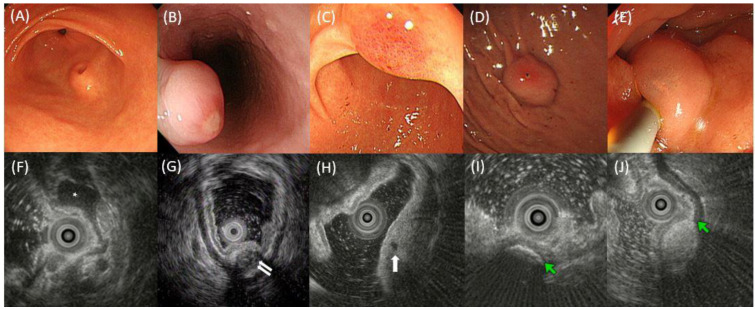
Examples of the endoscopic and EUS findings of SELs. (A) Umbilication or central dimpling, (B) erosion or ulcer, (C) erythema, (D) hemorrhagic spot, and (E) translucidity. (F) EUS image showing a homogeneous hypoechoic SEL (asterisk) with a distinct border; (G) EUS image showing an SEL involving muscle propria layer (white arrows) with heterogeneity, mixed echogenicity, indistinct border, and deep attenuation; (H) EUS image showing anechoic foci (white arrow); (I) EUS image showing hyperechoic foci (green arrow) with acoustic shadowing that suggests calcification; (J) EUS image showing an SEL involving submucosal layer (green arrow indicates hyperechoic submucosal layer) with hyperechoic echogenicity, homogeneity, and distinct border. EUS, endoscopic ultrasonography; SEL, subepithelial lesion.
